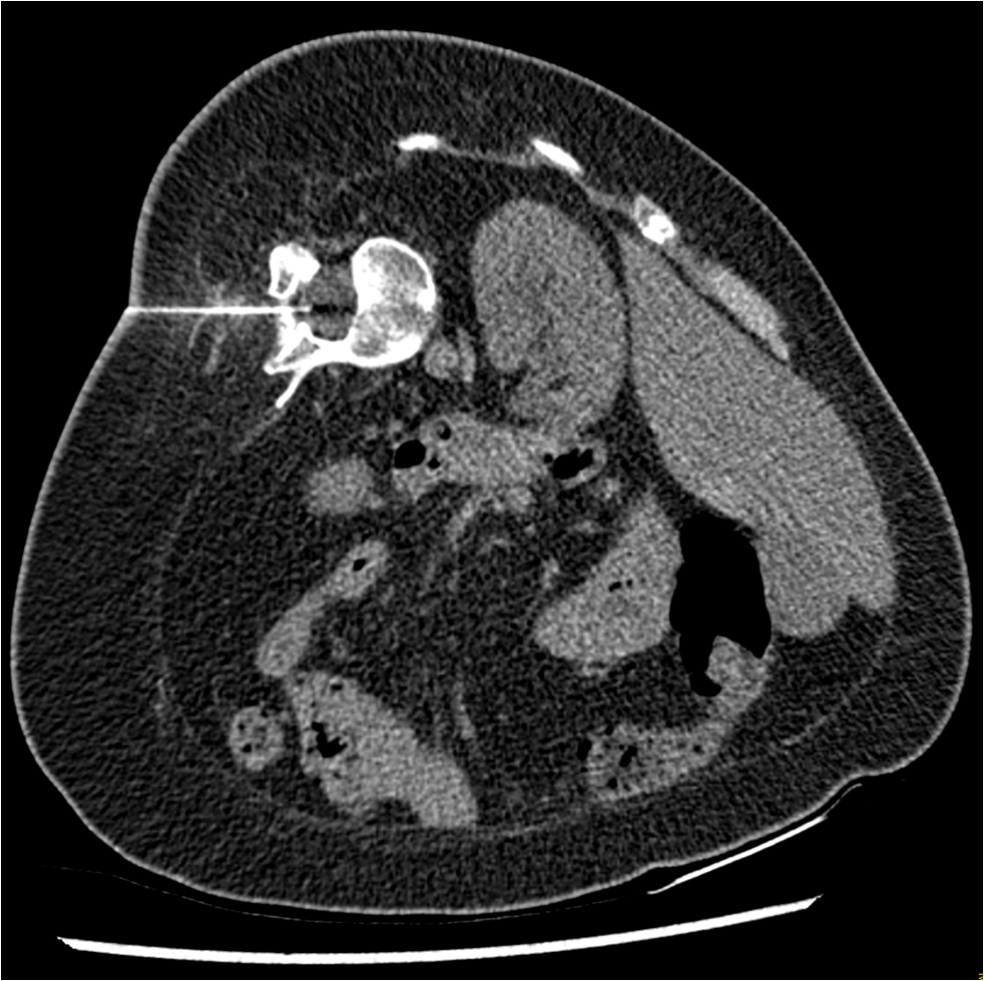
CT-guided intrathecal nusinersen injection—standard protocol
